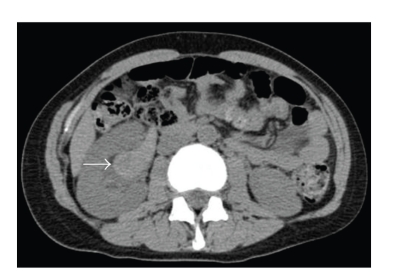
Axial CT image indicating that the right renal pelvis was filled with hematoma (arrow).
